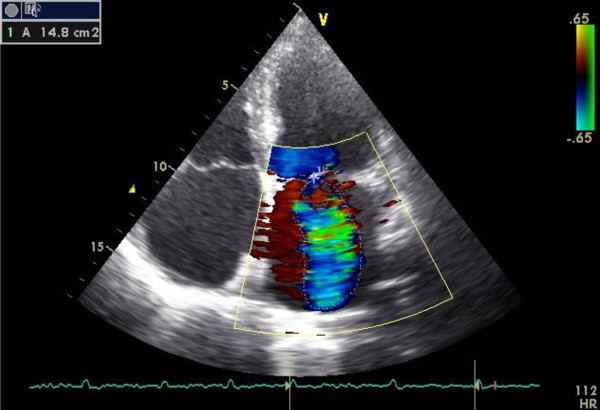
Moderate to severe mitral regurgitation (red) seen in echocardiography (parasternal view).
